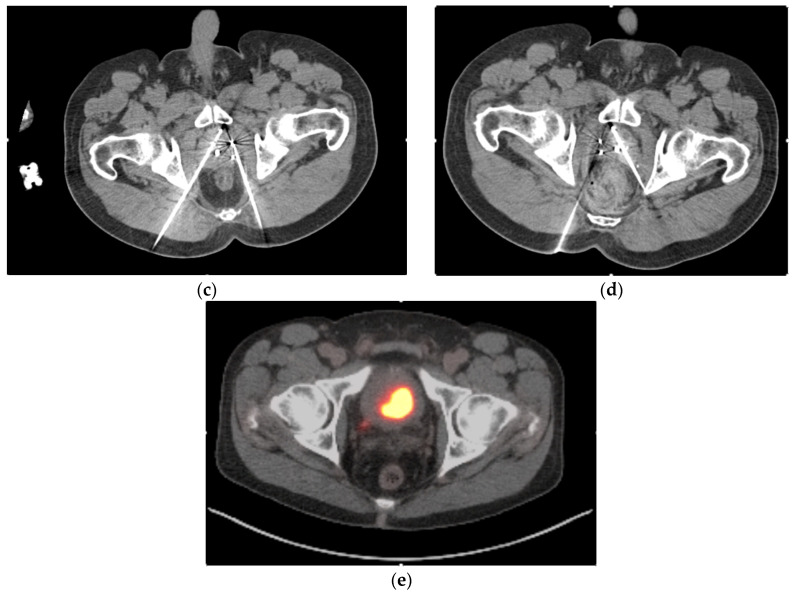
Case example of focal CT-guided cryoablation of locally recurrent prostate cancer. (a) Fused axial images of a fluciclovine-flourine-18-labeled SPECT/CT showing a focus of avidity along the right peripheral zone apex, correlating with local prostate cancer recurrence. (b) Axial contrast-enhanced T1-weighted MRI subtraction image showing the enhancement of locally recurrent prostate cancer within the right peripheral zone apex correlating with area of avidity (a). (c) Axial CT images showing transgluteal IcePearl cryoablation needle within the target lesion within the right hemi-prostate and placement of perirectal hydrodissection needle. (d) Transgluteal cryoablation probe into the prostate target lesion after instillation of 2% contrast:0.9% saline solution within the perirectal region to safely separate critical structures for cryoablation. (e) Fused axial images of the post-ablation 68Ga-PSMA-11 PET-CT showing no residual avidity along the right peripheral zone apex.
